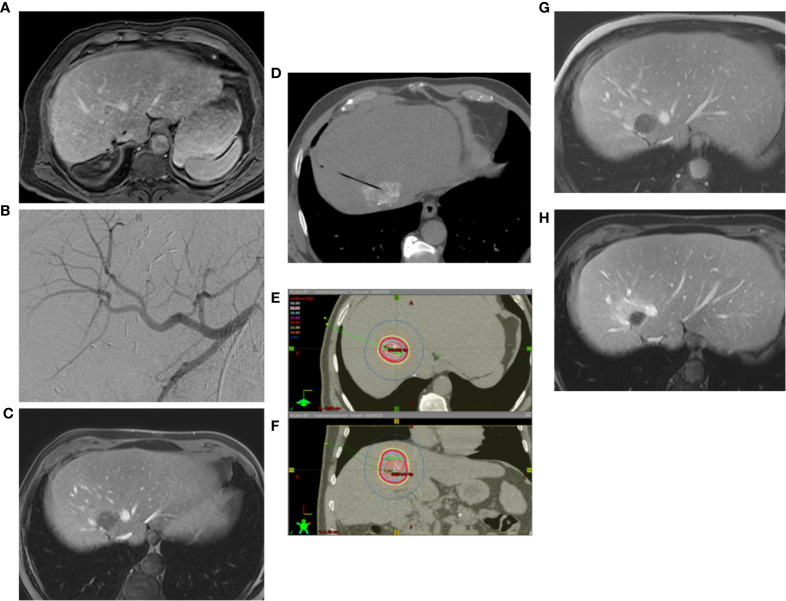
(A) Renewed solitary CRC-metastasis after multiple surgical metastasectomies, sometimes with complicated postoperative course until sepsis. (B) The difficultly located new metastasis between the hepatic veins in S VIII has been initially transartelically chemoembolized (TACE). (C) Post-interventional contrast-enhanced MRI shows subtotal devascularisation of the target lesion. (D) Interstitial brachytherapy was performed sequentially. Lipiodol labelling from TACE was used for navigation of the brachytherapy applicator. (E) The image shows the isodose distribution in the axial plane. (F) Isodose distribution in the coronary plane. (G) contrast enhanced MRI reveals an excellent local tumor control after 3 months. (H) After 6 months the tumor cavity shrinks in time, there is still no recurrence, only perifocal postradiogenic changes.
